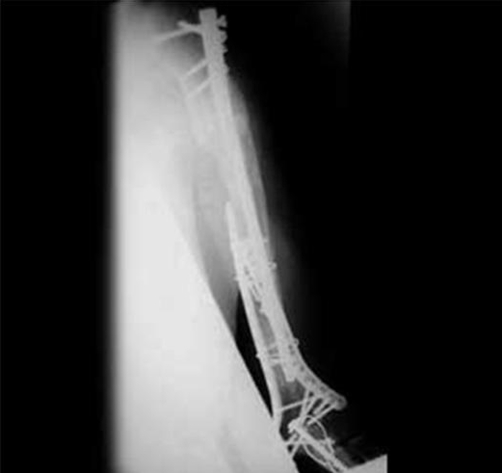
Lateral radiograph of left elbow post fixation with elbow in full extension.
